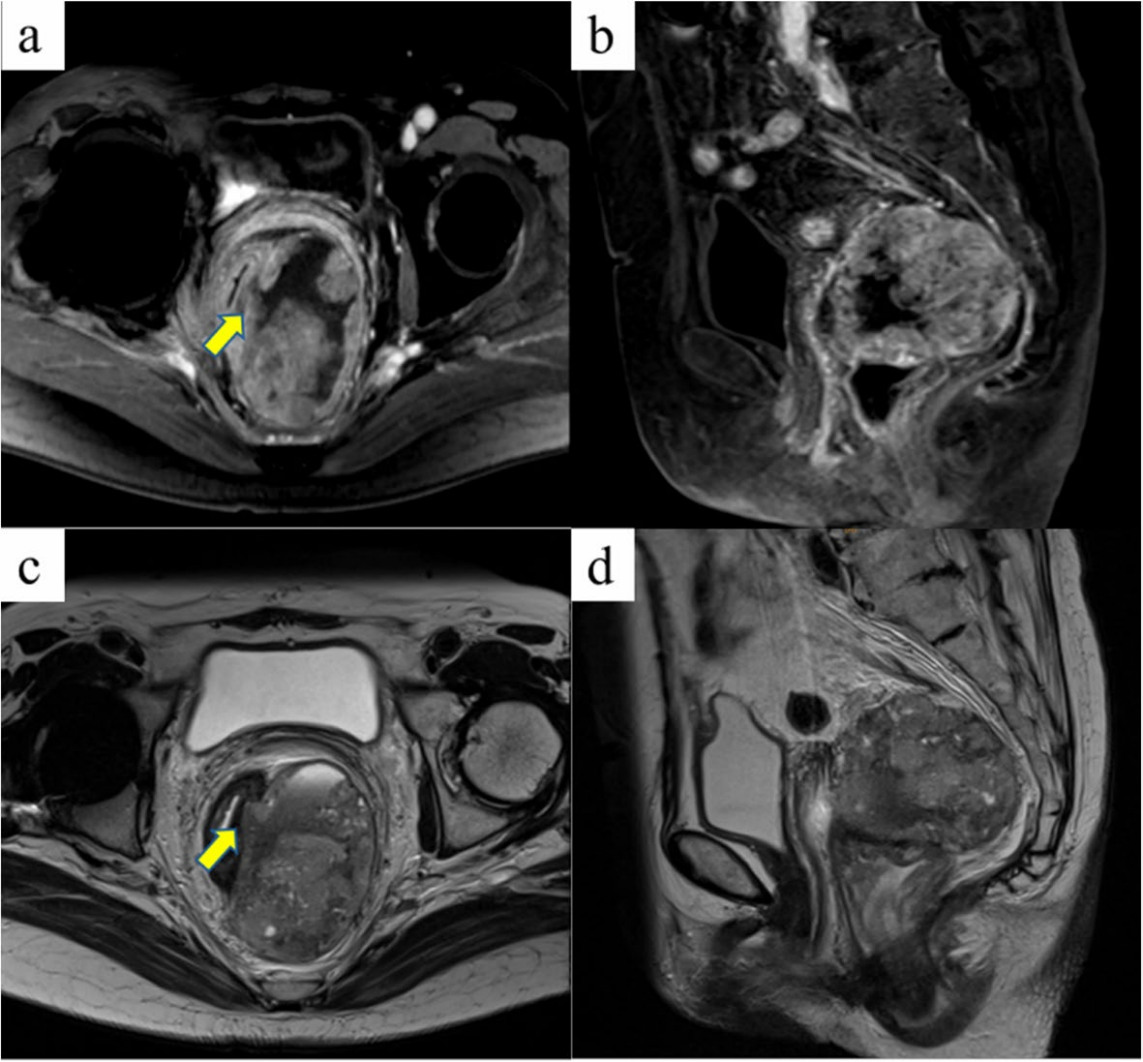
Contrast-enhanced pelvic magnetic resonance images. Coronal fat-suppressed T1-weighted (a), sagittal fat-suppressed T1-weighted (b), coronal T2-weighted (c), and sagittal T2-weighted images (d) are presented. The tumor was continuous with the muscular propria on the left side of the rectum (indicated by an arrow)
